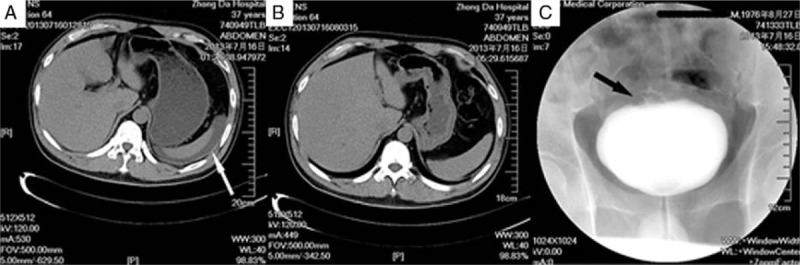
Imaging examination of the patient. (A) Axial computed tomography (CT) image showed a few of liquid dark areas around the spleen (white arrow); (B) CT recheck indicated the disappearance of the intraperitoneal fluids; and (C) cystography indicated a small amount of suspicious contrast agent leakage (black arrow).
